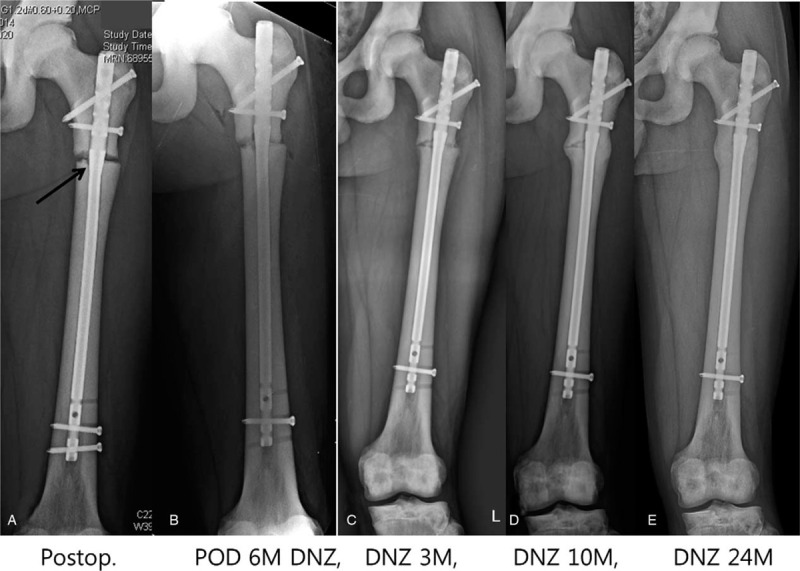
A Subtrochanteric fracture in a 26-year-old man with autosomal dominant type II osteopetrosis. A. A subtrochanteric fracture was treated with intramedullary nailing. However, a gap in the fracture site occurred because of contact between the transitional part of the nail and the distal fragment (arrow). B. Delayed union was evident at 6 months after the operation, and dynamization (DNZ) was performed. C. Callus formation was observed at 3 months after the DNZ. D. The fracture attained union 10 months after the DNZ. F. The final radiograph 24 months after the DNZ shows complete union and remodeling of the callus. POD, postoperative day.
